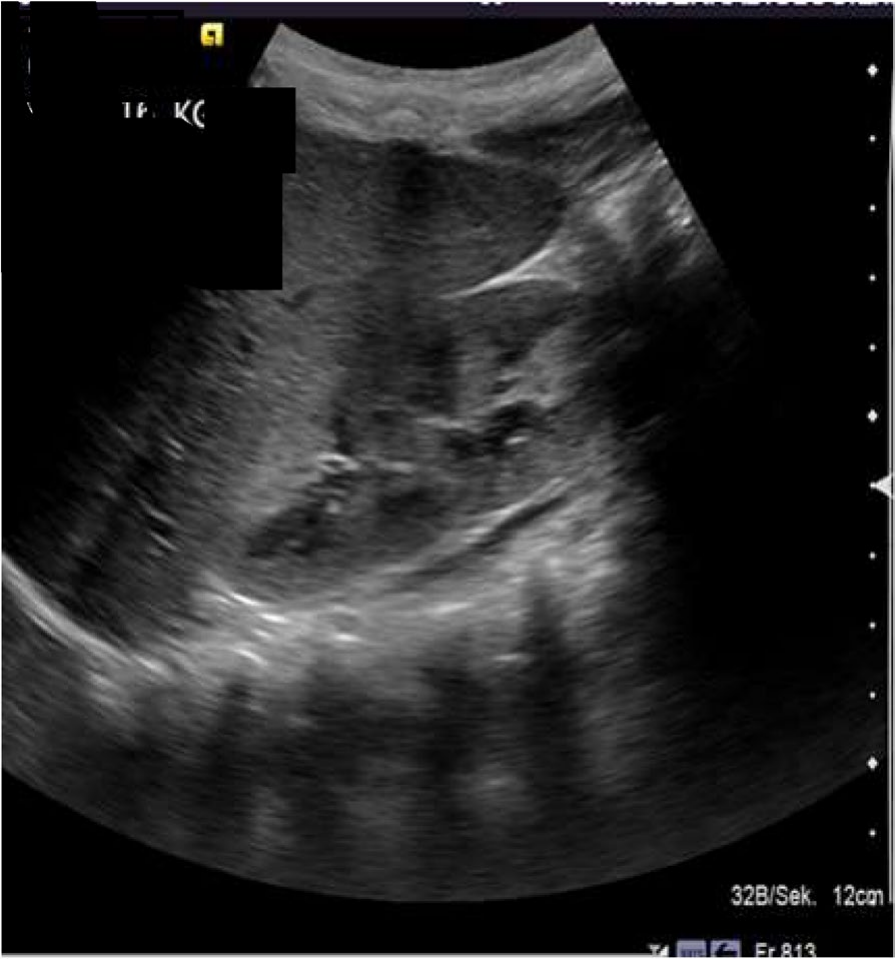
Renal ultrasound showing an enlarged hyperechogenic kidney. Otherwise, this was an age-appropriate abdominal sonogram
